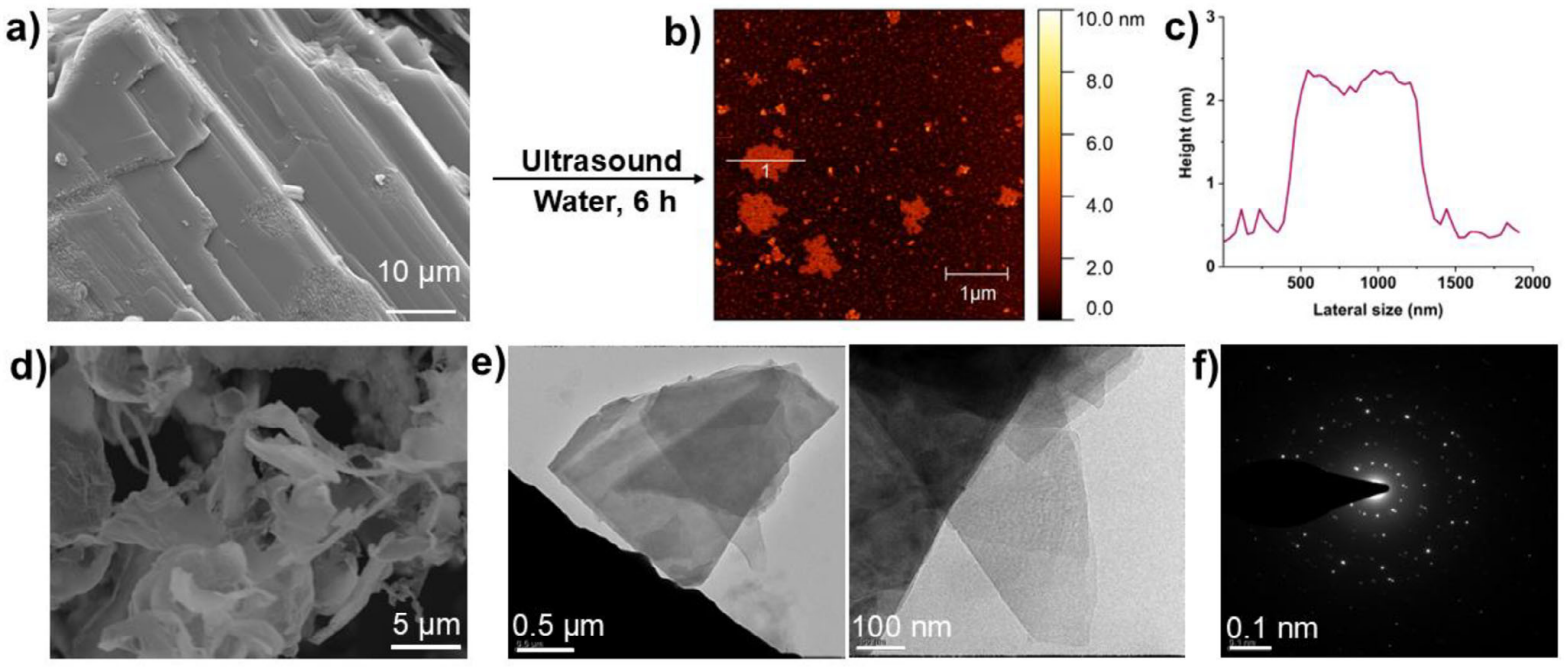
Ultrasonic liquid exfoliation of a) layered co‐crystal 1 in water medium to form b) nanosheet XON1. c) Height profile of the nanosheet. d) SEM image of the freeze‐dried XON1. e) TEM images and f) Electron diffraction of nanosheet XON1.
